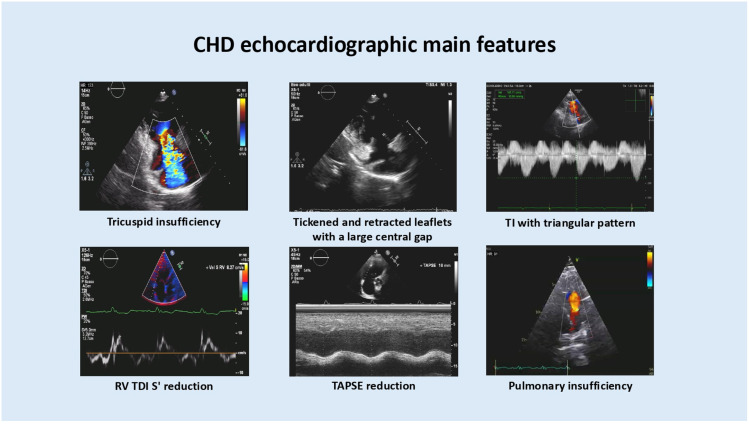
Main echocardiographic features observed in patients with carcinoid heart disease.
